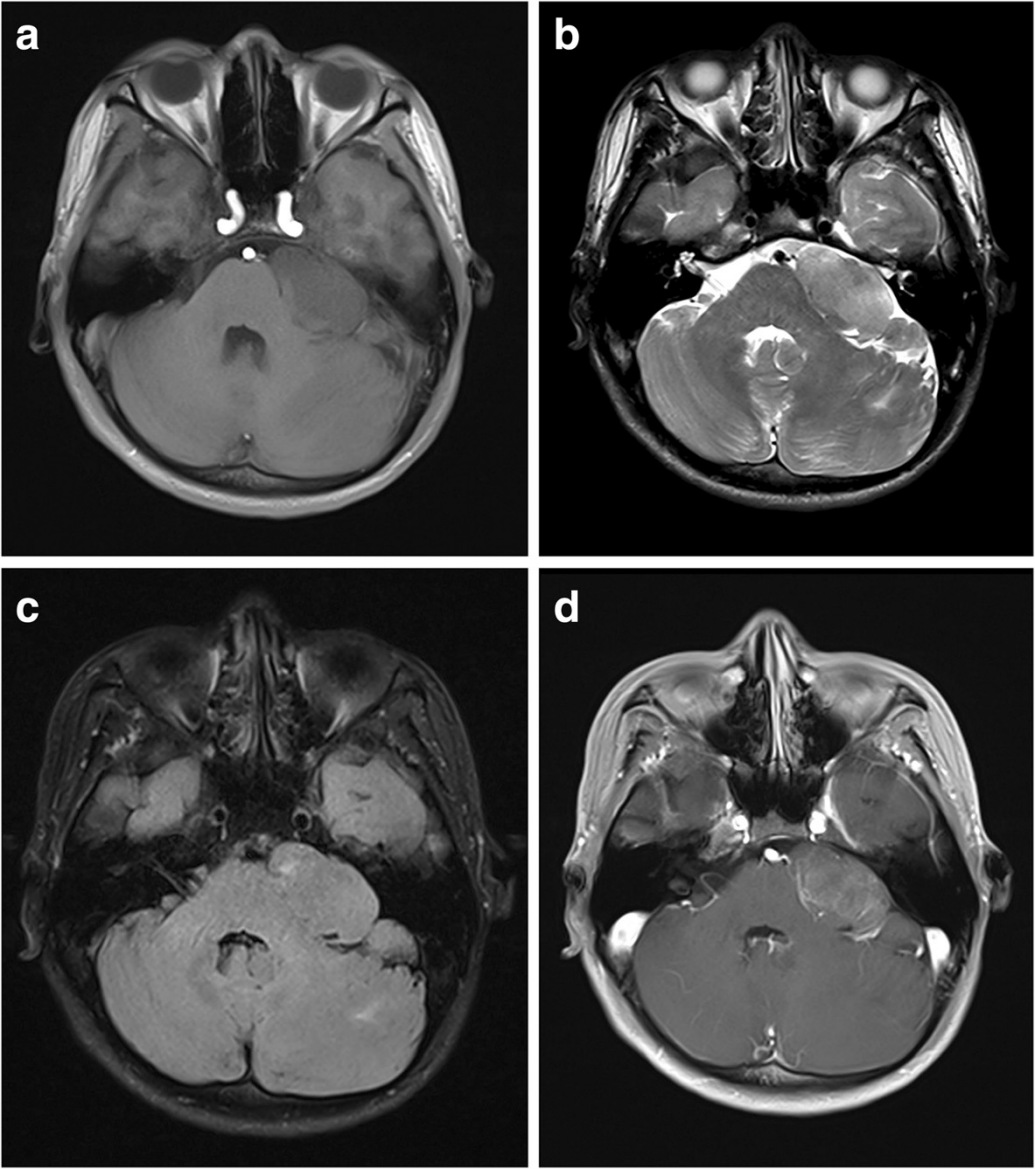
Conventional MRI of trigeminal ganglioneuroma. The tumor was homogeneously hypointense on T1WI (a),and mix iso-hyperintense on T2WI (b) and T2WI-Flair (c), it showed heterogeneous moderate enhancement on enhanced imaging (d)
